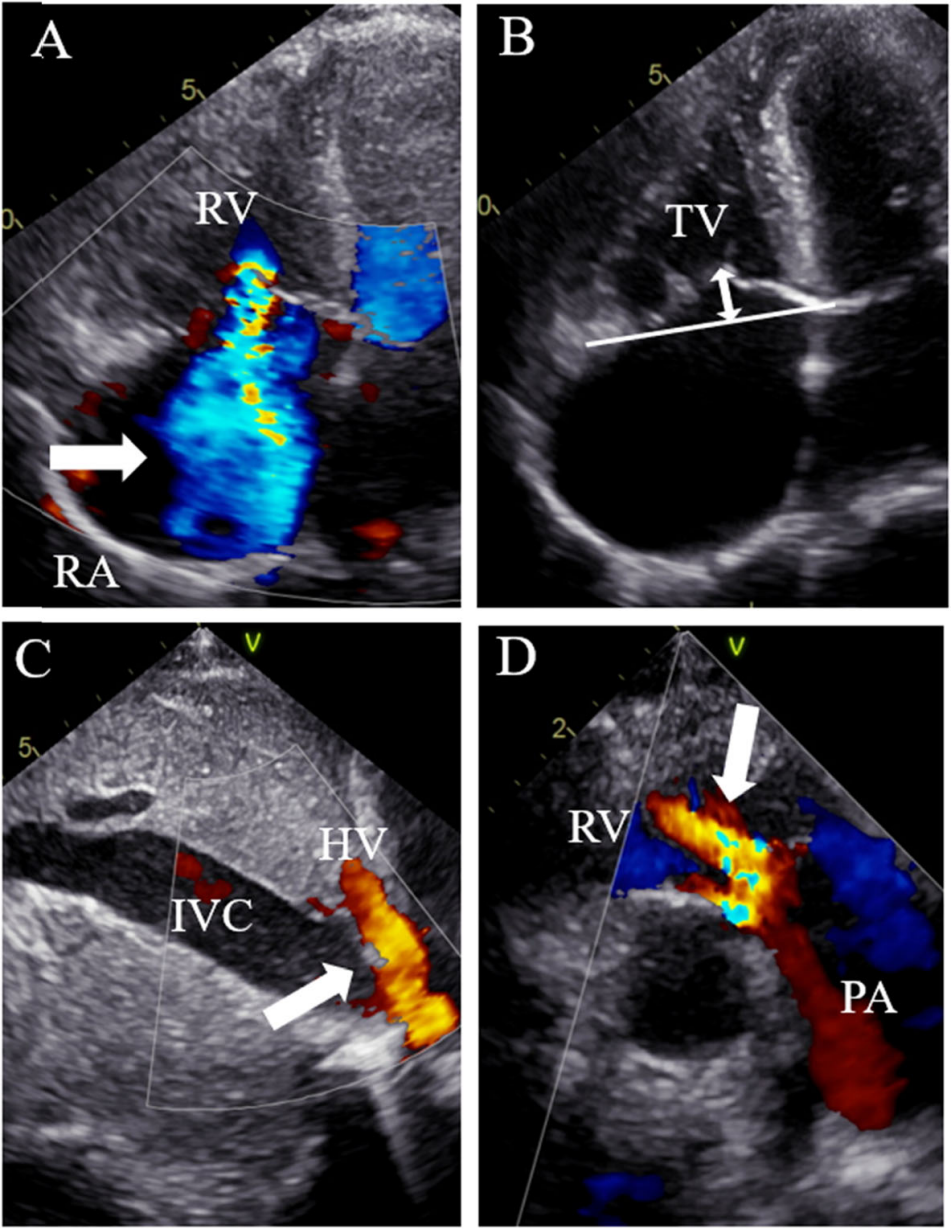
Preoperative transthoracic echocardiography. a: Massive tricuspid regurgitation with wide vena contracta. b: Extensive tethering height (double headed arrow). c: Systolic hepatic vein flow reversal. d: Moderate pulmonary regurgitation. RV, right ventricle; RA, right atrium; TV, tricuspid valve; IVC, inferior vena cava; HV, hepatic vein; PA, pulmonary artery
